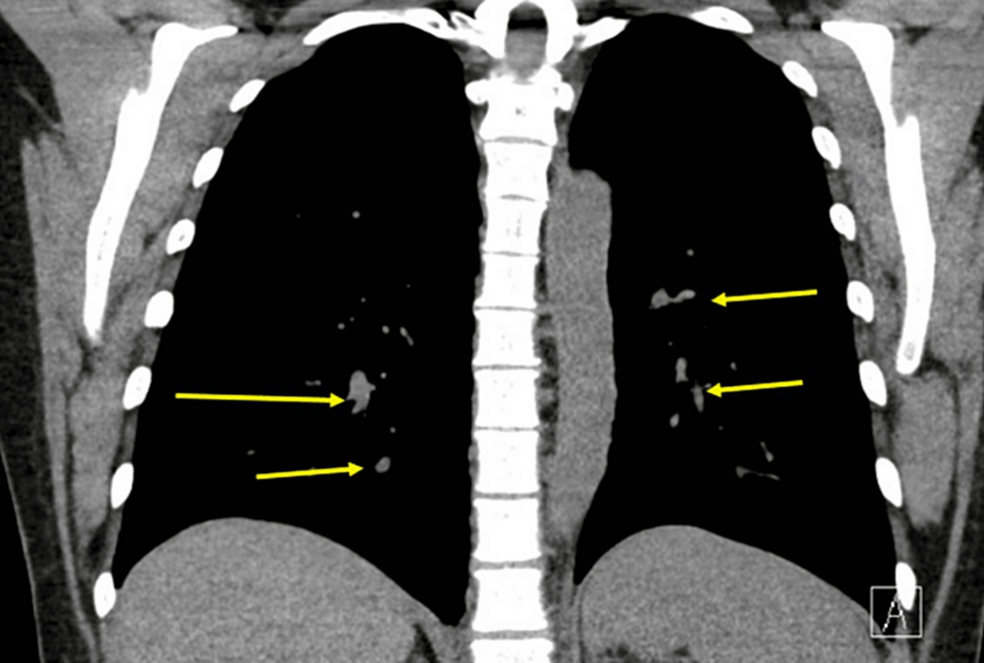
CT chest showing possibly metastatic pulmonary nodules in right and left lower lobes Pulmonary nodules in the left upper lobe (3 mm), right lower lobe (3 mm), right lower lobe (6 mm), and left lower lobe (4-5 mm).
